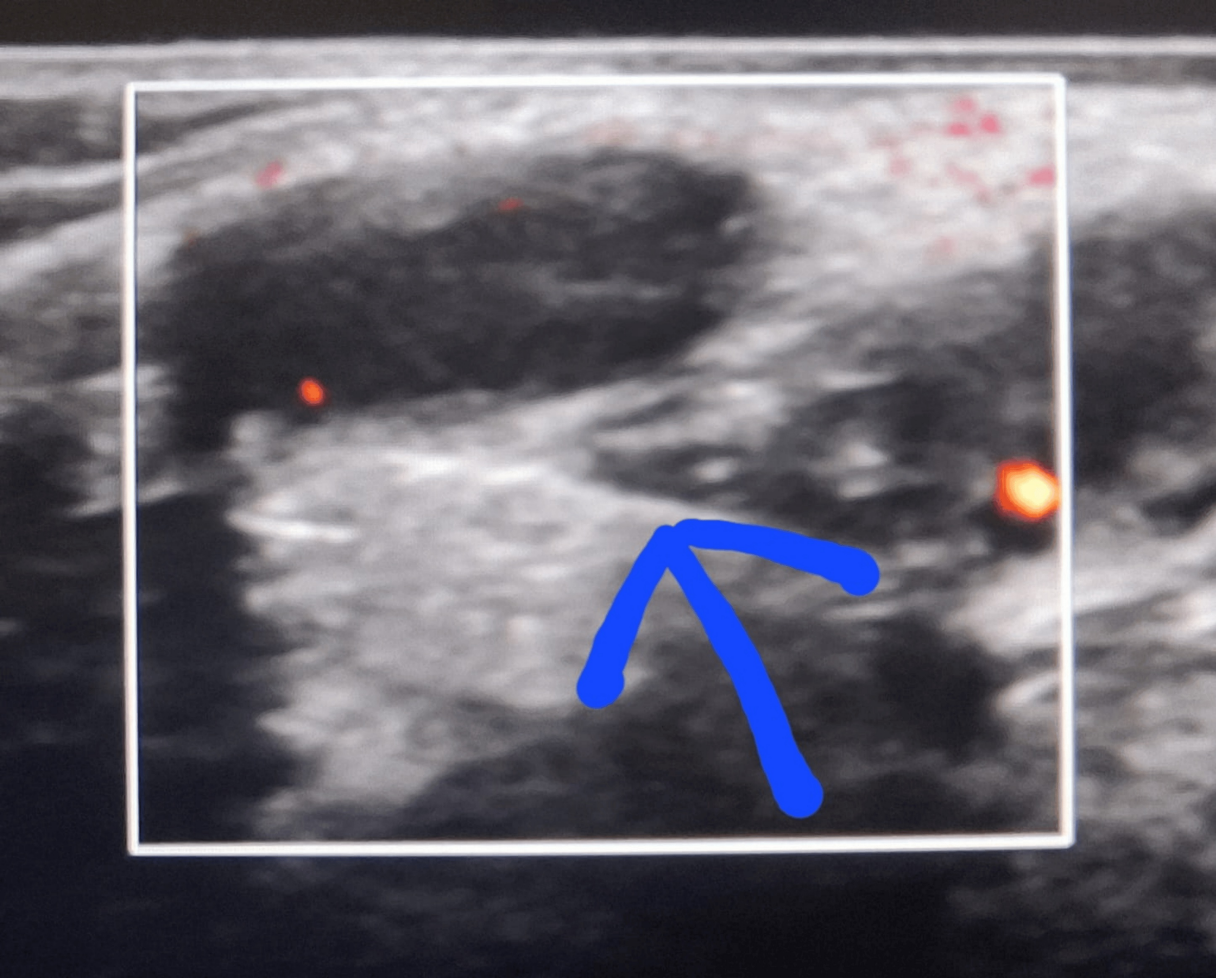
Ultrasound of the right inguinoscrotal region The arrow shows the defect in the deep inguinal ring.
